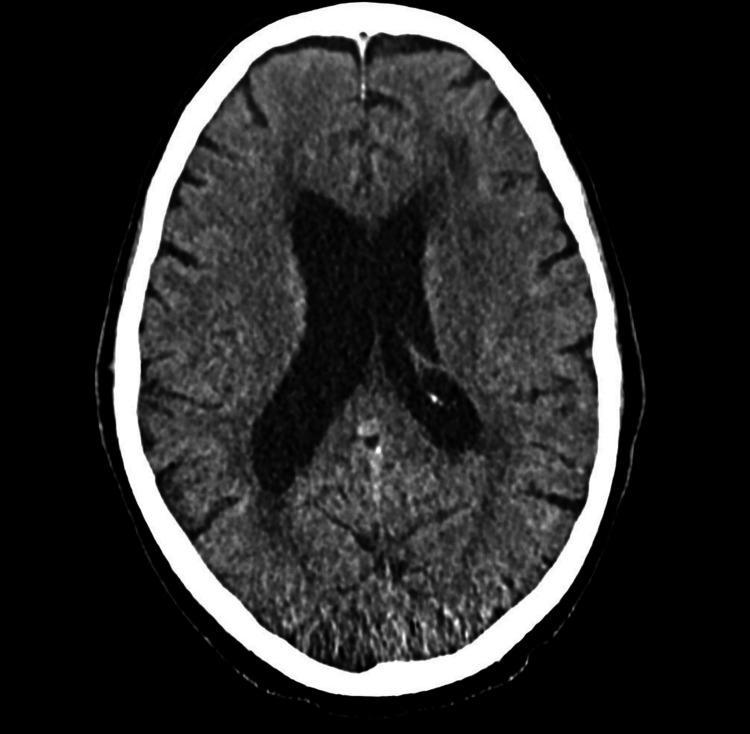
Head CT showing no acute intracranial findings.
